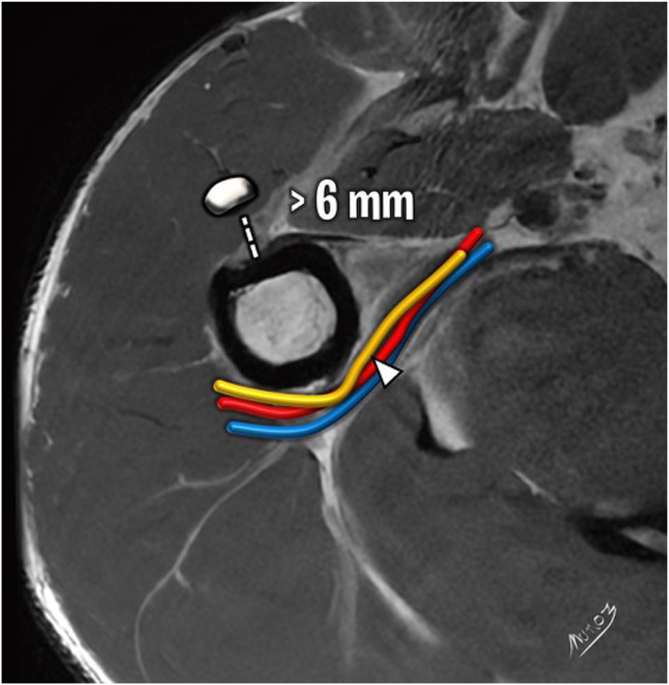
LHBT-groove distance sign (dashed line) is shown in an axial view of a T1-weighted right shoulder MRI. The axillary nerve is indicated with a white arrowhead. The lines represent the axillary neurovascular bundle comprised of the axillary artery (red line), vein (blue line), and nerve (yellow line). (LHBT, long head biceps tendon; MRI, magnetic resonance imaging.)
